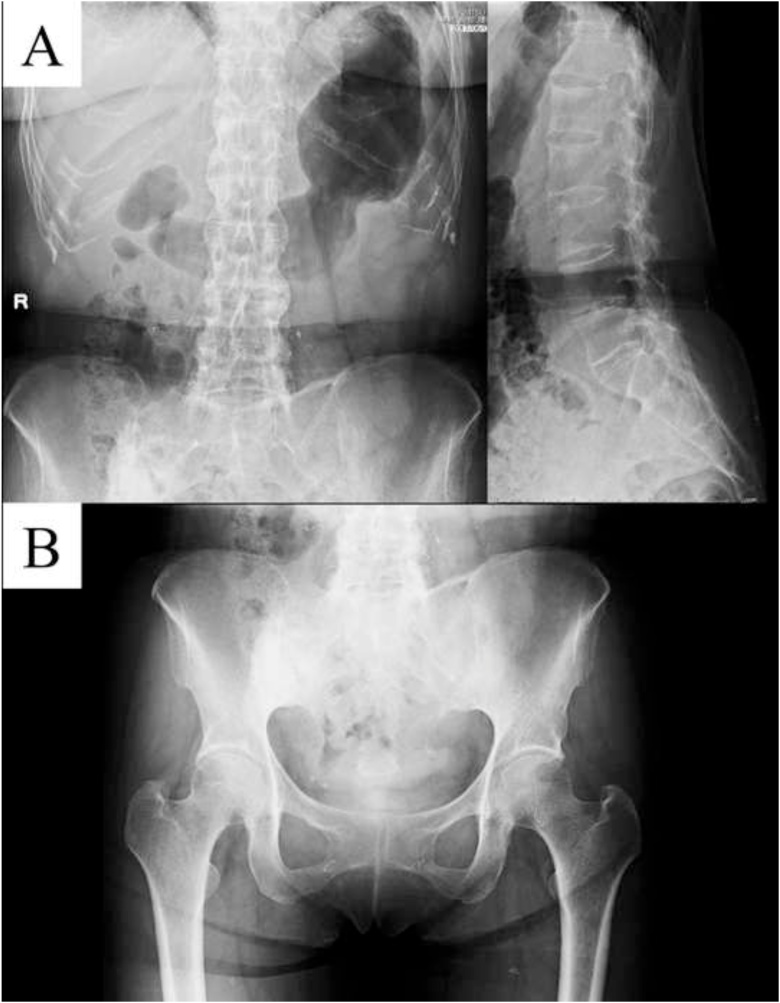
(A) Anteroposterior and lateral X-ray image of the lumbar spine, and (B) anteroposterior X-ray image of the pelvis.
